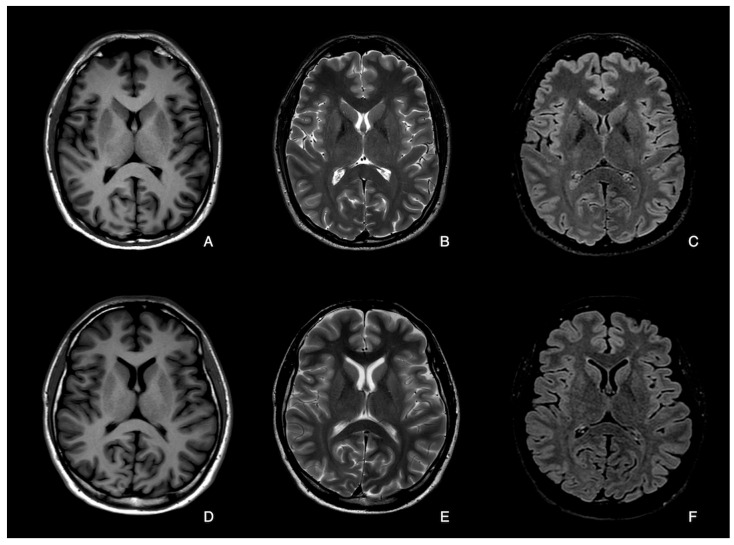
Comparison between Compressed-SENSE and No Compressed-SENSE sequences in two healthy subjects, both 28 years old. Upper panel: Compressed-SENSE T1-Turbo Spin Echo (TSE) (A), Compressed-SENSE T2-TSE (B) and Compressed-SENSE 3D-T2-FLAIR (C). Lower panel: T1-TSE (D), T2-TSE (E) and 3D-T2-FLAIR (F).
